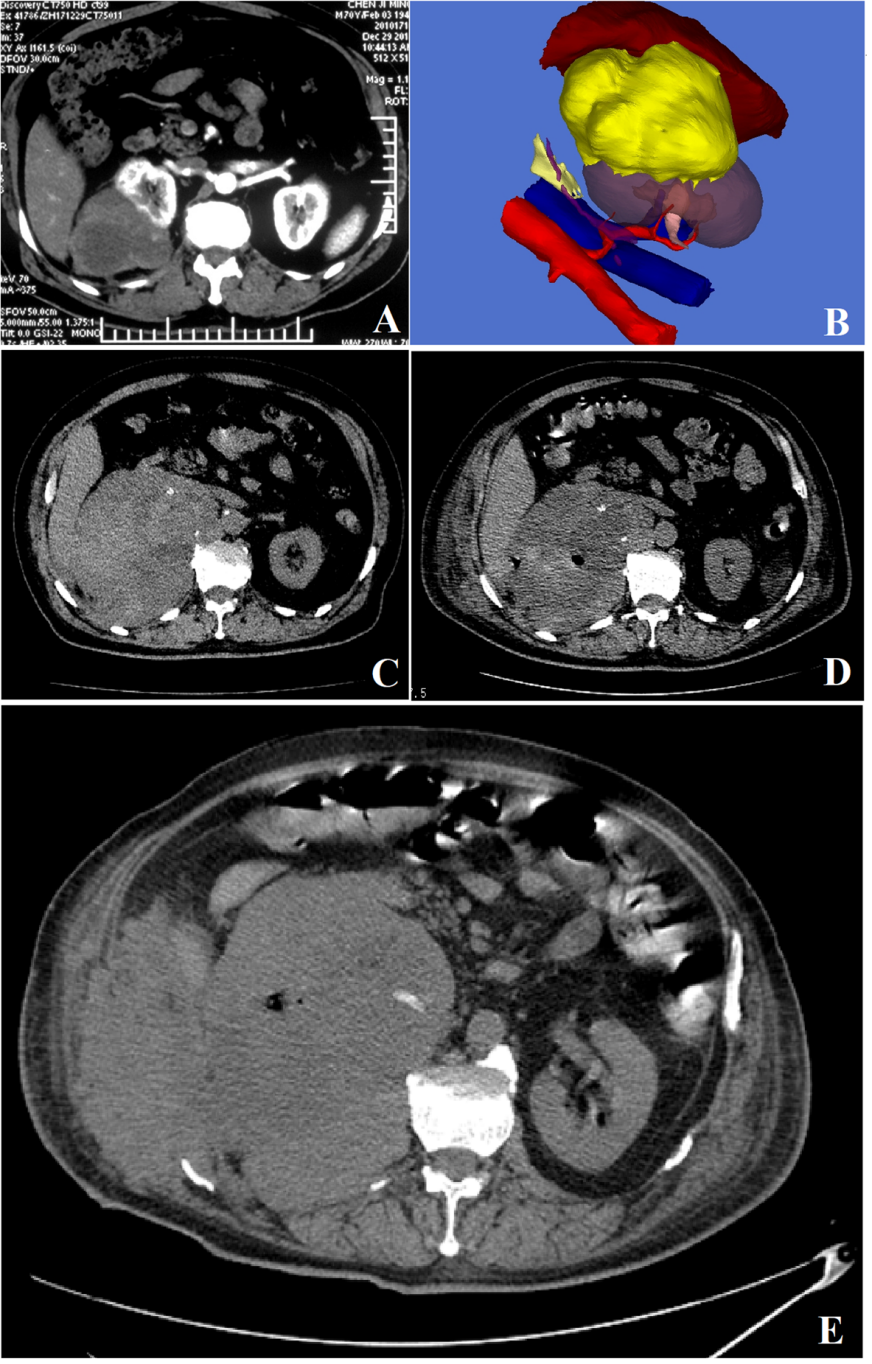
a The primary CT findings when admission showed the right renal mass with the size of 13.0 × 10.0 × 8.0 cm. b The reconstructed three-dimensional digital model (3D-DM) marked by using different colors (yellow color: the renal mass). c The rechecked CT findings when readmission showed a right retroperitoneal hematoma with the size of 16.3 × 13.2 × 10.4 cm. d The rechecked CT scanning result after the percutaneous puncturing and drainage showed no significant shrinkage for the retroperitoneal hematoma. e The day before his clinical death, the rechecked CT scanning images showed that the rapid retroperitoneal cavity metastasis with obvious sign of puncturing track implantation
